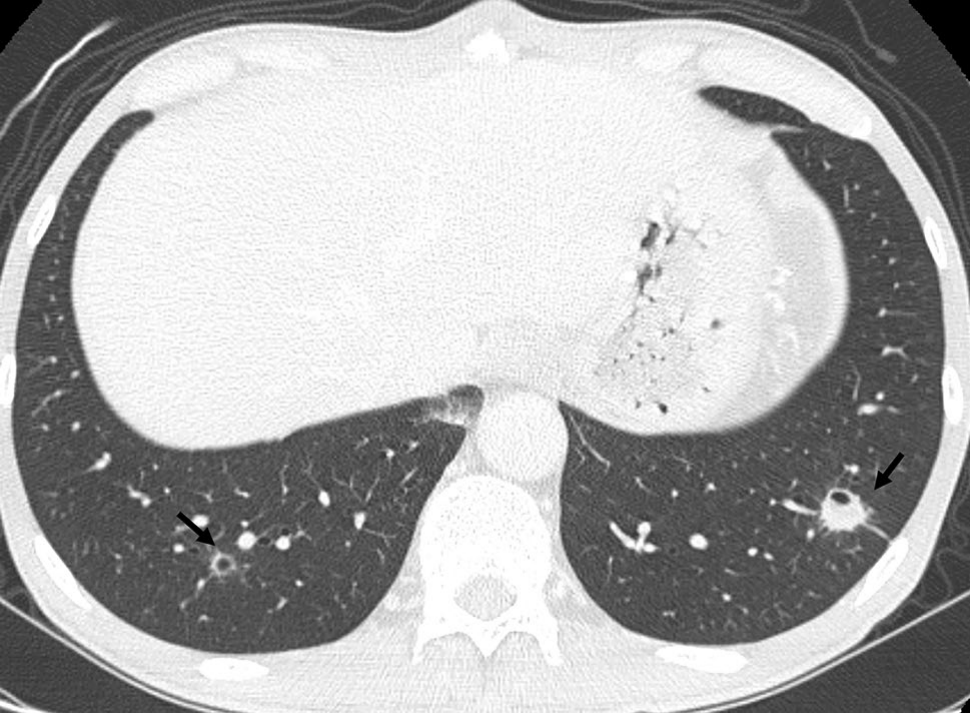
Septic embolism due to methicillin-sensitive Staphylococcus aureus (MSSA). A man in his 40 s developed a septic embolism secondary to a tonsillar abscess caused by MSSA. Chest CT revealed cavitary nodules in the peripheral regions of both lower lung lobes. A cavity in the right lower lobe shows an air-fluid level
